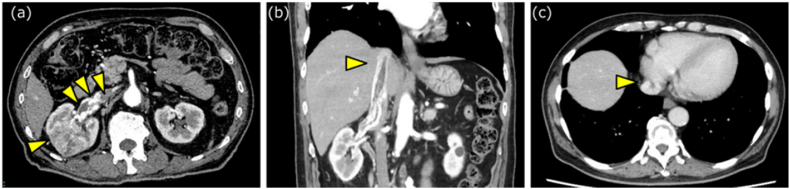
Contrast-enhanced computed tomography (a) Tumor with heterogeneous enhancement in the early contrast phase (arrow) and tumor thrombus extending from the right renal vein into the inferior vena cava (triangle). (b–c) The tip of the tumor thrombus extends beyond the diaphragm, approaching the vicinity of the right atrium.
